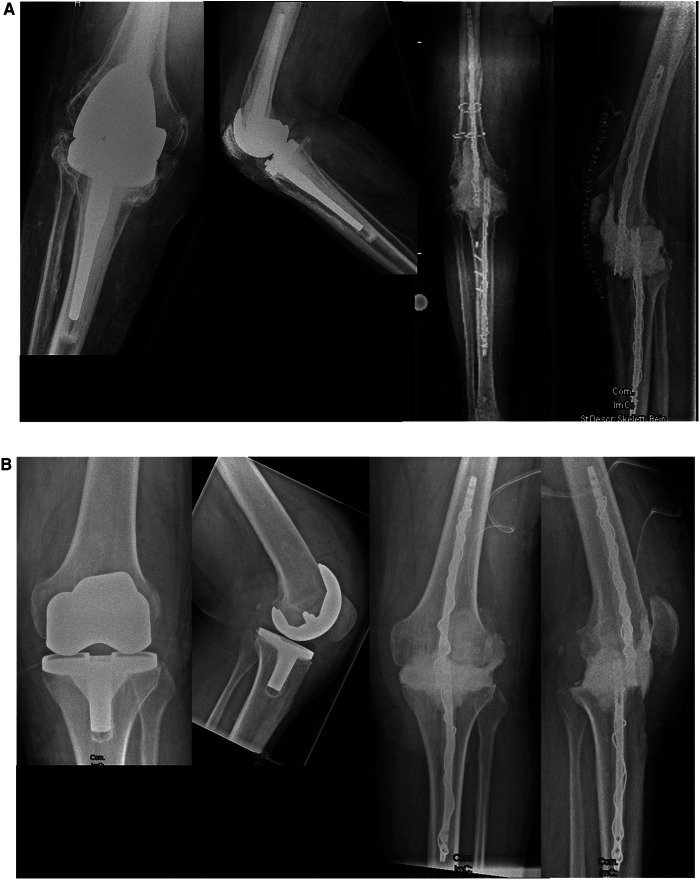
Pre-operative x-rays of (A) an infected rotating hinge prosthesis and (B) an infected bicondylar surface replacement prosthesis are shown in the left panel. Post-operative images after explantation, debridement, and temporary arthrodesis are shown in the right panel.
